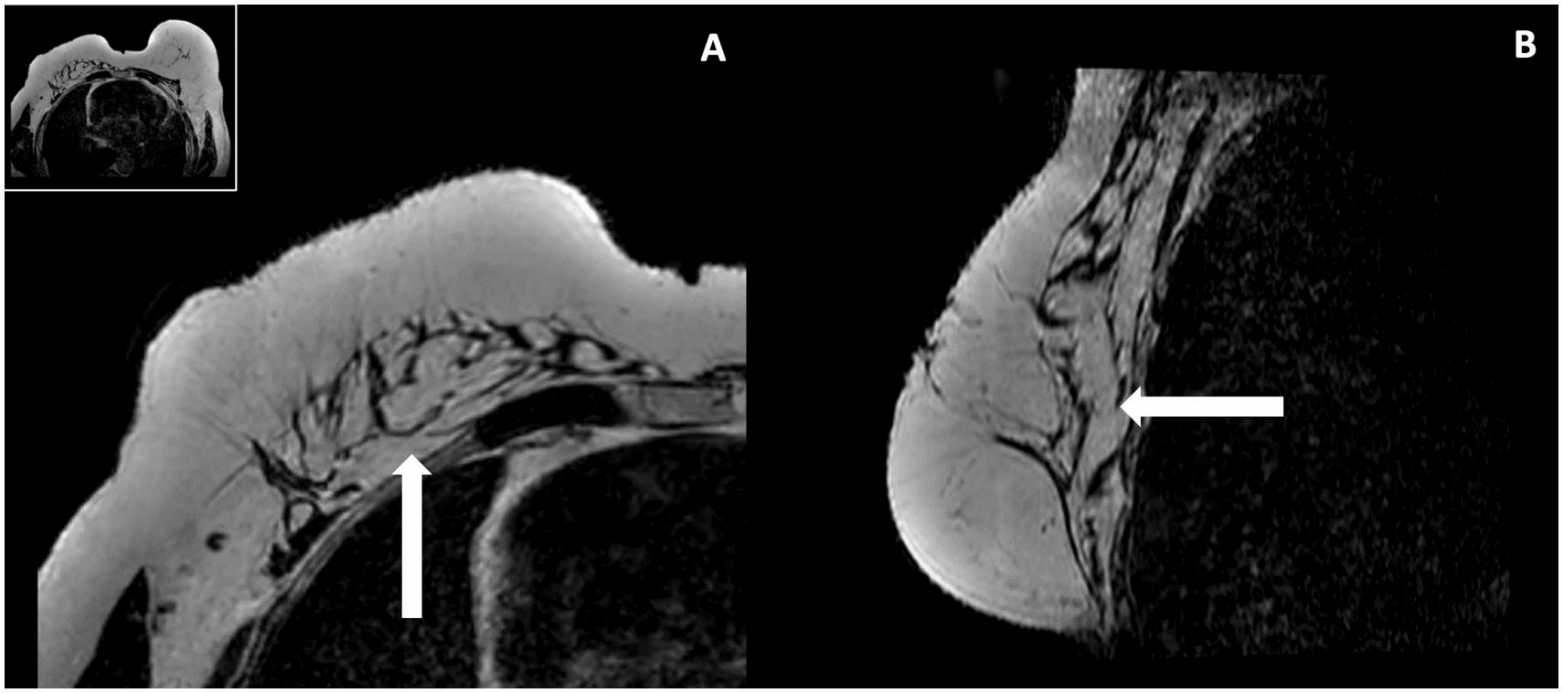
Radiographic change of the pectoral muscle after finishing a total breast reconstruction with AFT. This is a beast MRI of a 68-year-old patient that was 2 years after her last bilateral AFT procedure. (A) The axial T2W image of the right reconstructed breast with fat depositions within the pectoral muscle. (B) The corresponding sagittal view. Abbreviations: AFT = autologous fat transfer; MRI = magnetic resonance imaging; T2W = T2 weighted.
